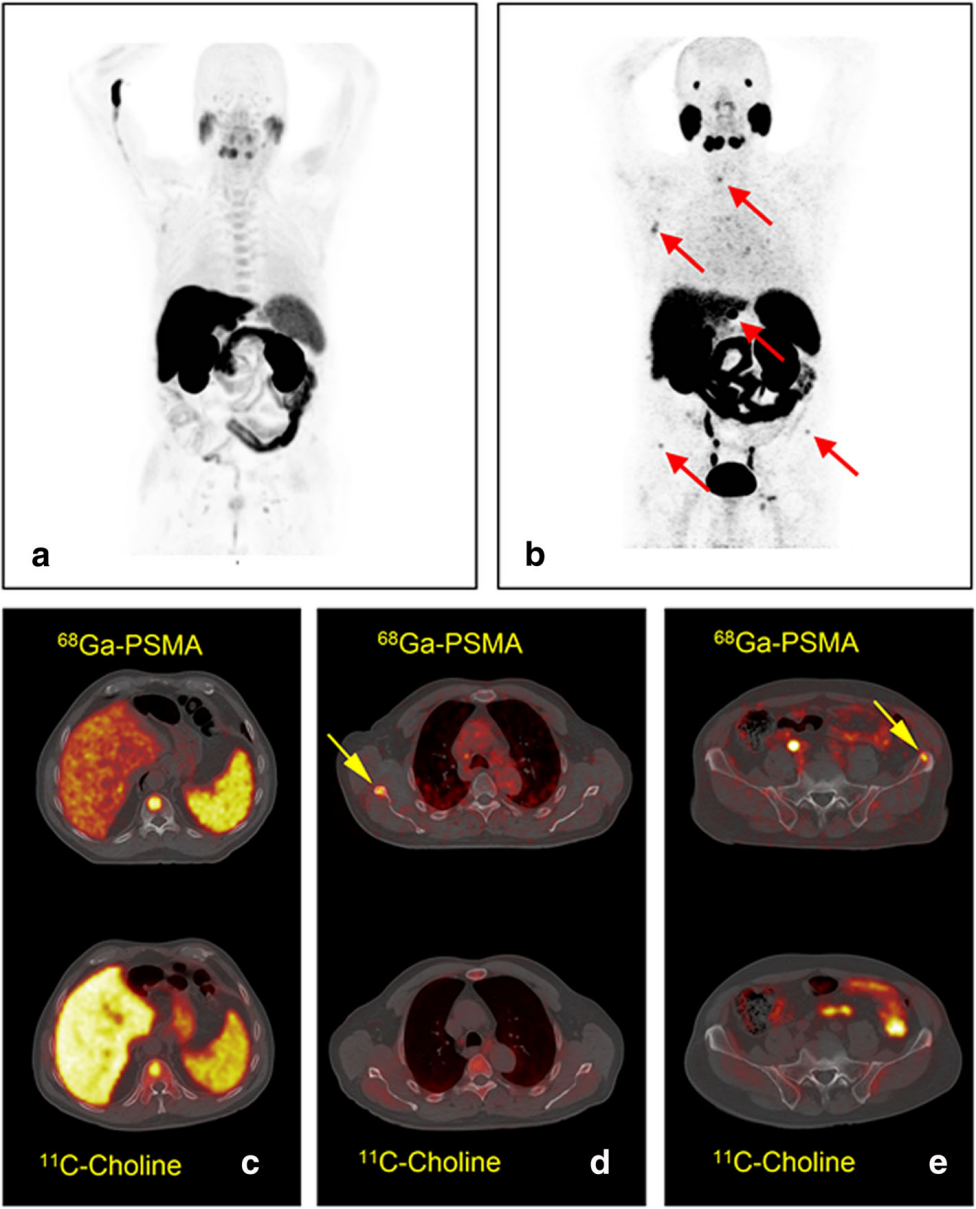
Patient 1. A 67-year-old patient treated with radiotherapy and hormonotherapy with PSA relapse (PSA level 8.7 ng/ml). MIP images with bone metastases (red arrows), scanned with ^11^C-Choline (a) and with ^68^Ga-PSMA (b). Metastasis in T11 demonstrated with both tracers (c). Right scapula (d) and left iliac crest (e) focal lesions demonstrated only with ^68^Ga-PSMA (yellow arrows)
